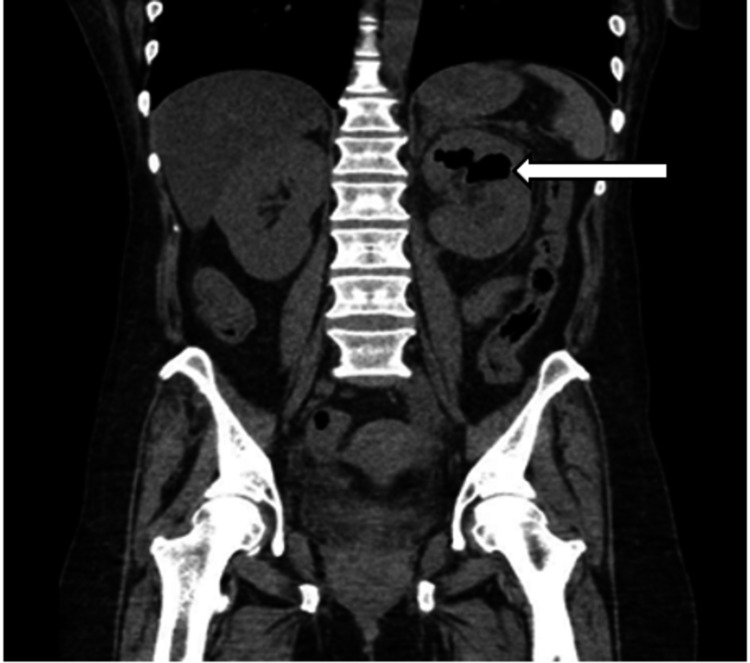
CT the abdomen and pelvis without contrast The white arrow demonstrates left hydronephrosis with gas within the left renal collecting system with possible extension into renal parenchyma.
